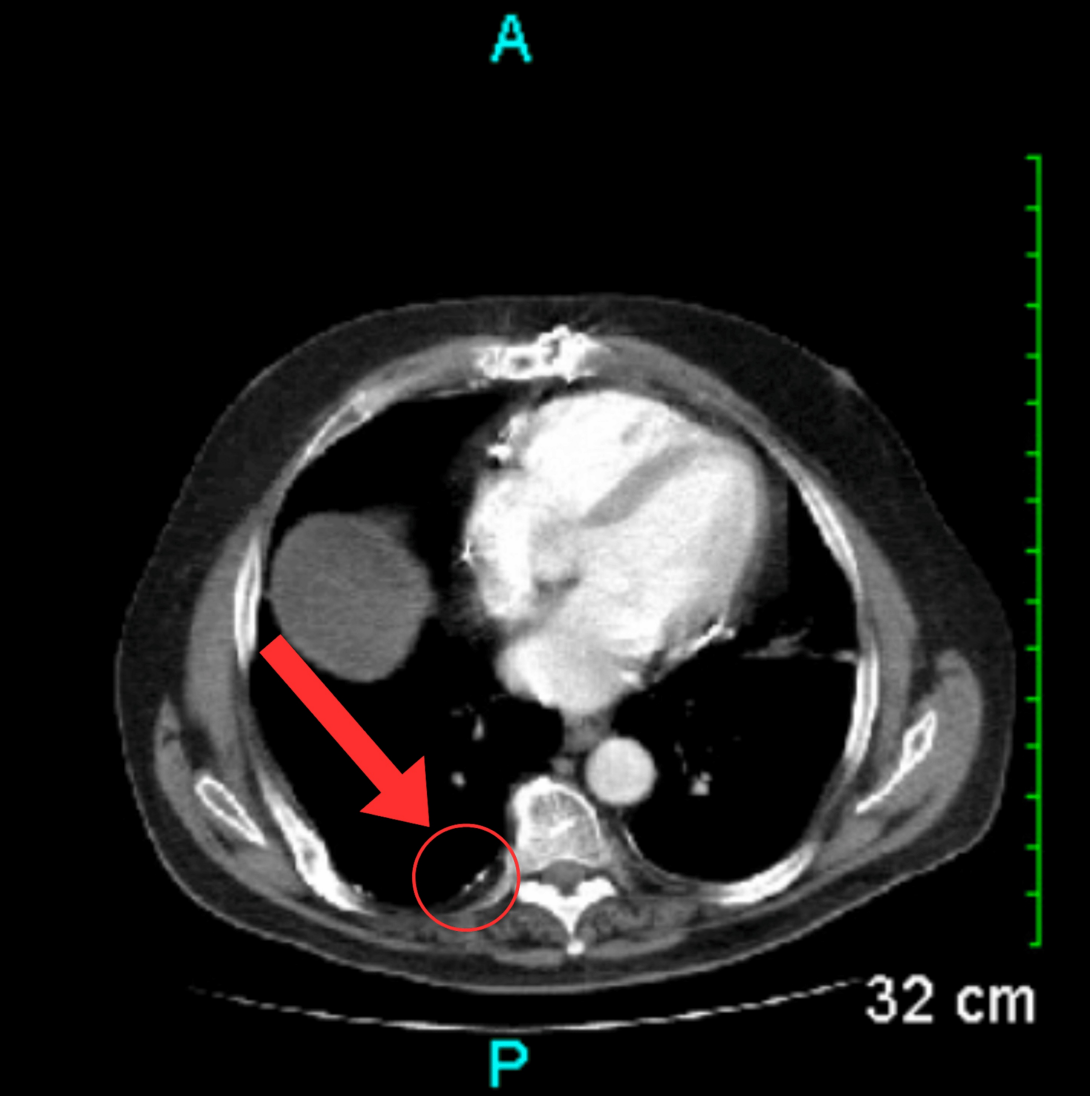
CT scan showing mild pleural calcifications posteriorly in the right hemithorax and over the posterior right hemidiaphragm (red arrow and circle). CT: Computed tomography
